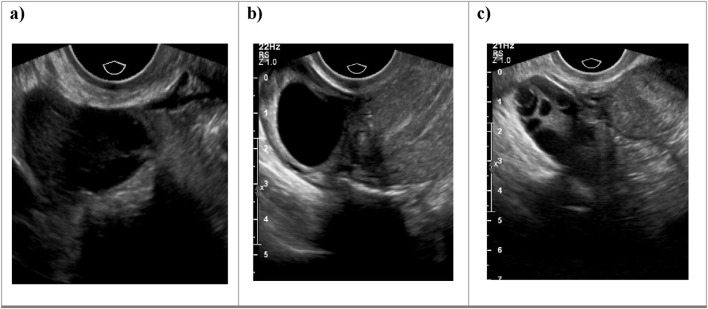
Sample ultrasound images from the dataset, representing the three classes: (a) Normal, (b) Dominant Follicle, and (c) Polycystic Ovary Syndrome (PCO). These examples highlight the visual differences between the classes used for muticlass classification.
